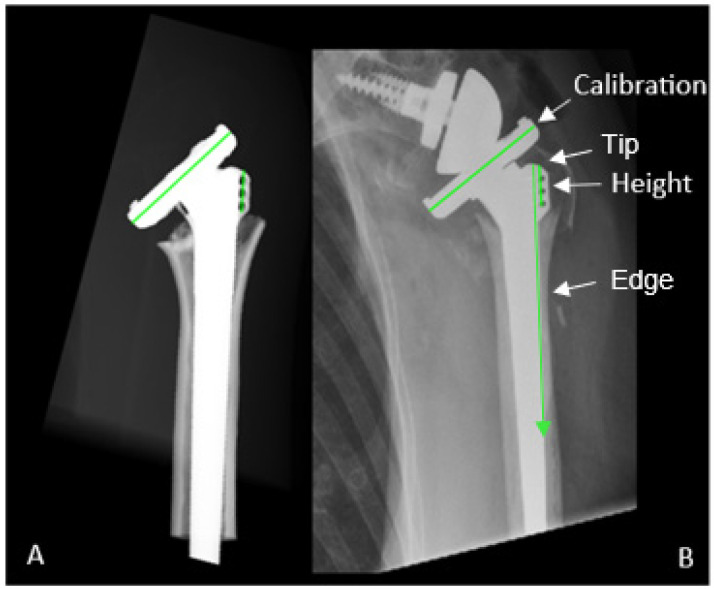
Humeral stem height measurement, calibrated with measurement of humeral tray (see green lines): (A) DRR of VSP; (B) postoperative radiograph.
